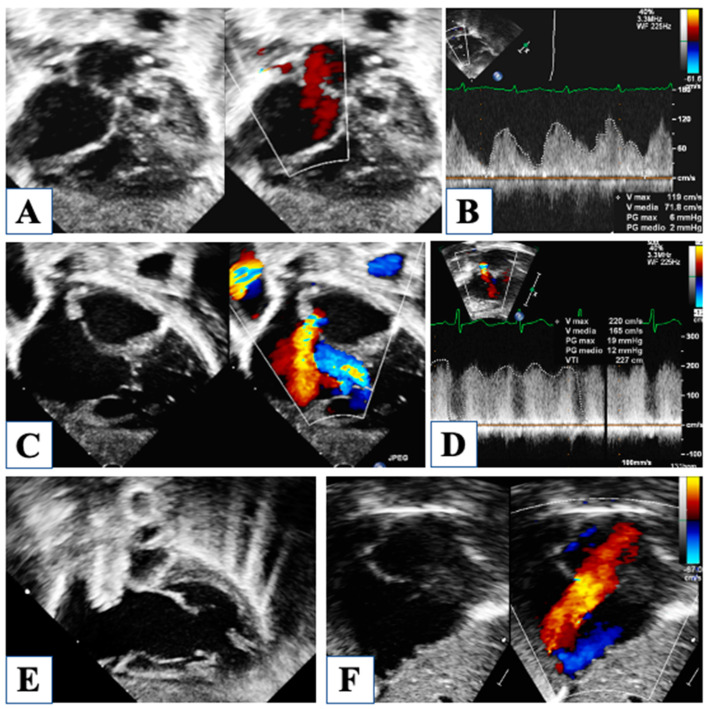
Atrial septal defect with malaligned septum primum and no restrictive flow (Panel (A)). Continous wave Doppler showing a low mean gradient of 2 mmHg through the atrial septum (Panel (B)). Unrestrictive ostium secundum atrial septal defect (Panel (C)). Critically small atrial septal communication with aliasing by color-Doppler (Panel (D)) and high mean gradient of 12 mmHg (Panel (E)). Successful stenting of the restrictive atrial defect (Panel (F)).
